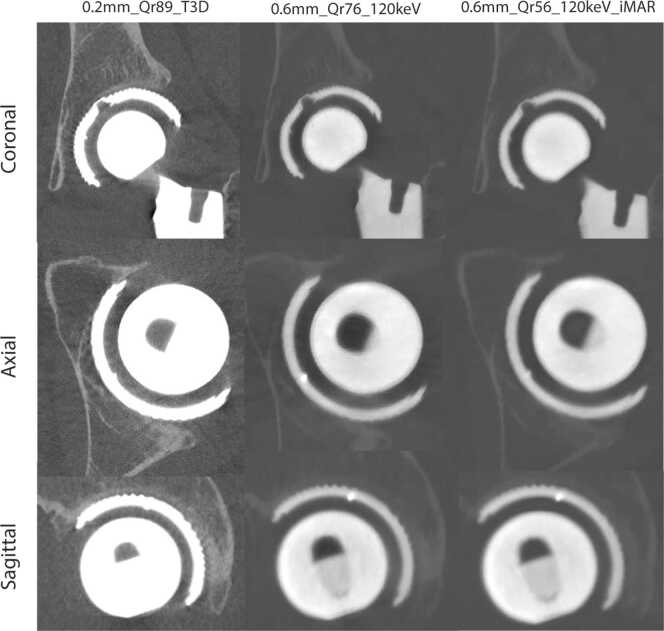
CT reconstructions used in the study showing an acetabular cup implant in different planes. The data was reconstructed in three ways: the left column shows the 0.2 mm Qr89 reconstructions, the middle column shows the 0.6 mm Qr76 and the column at the right shows the 0.6 mm Qr56_iMAR reconstructions. Window level and window center was 7500/2000 for all images.
